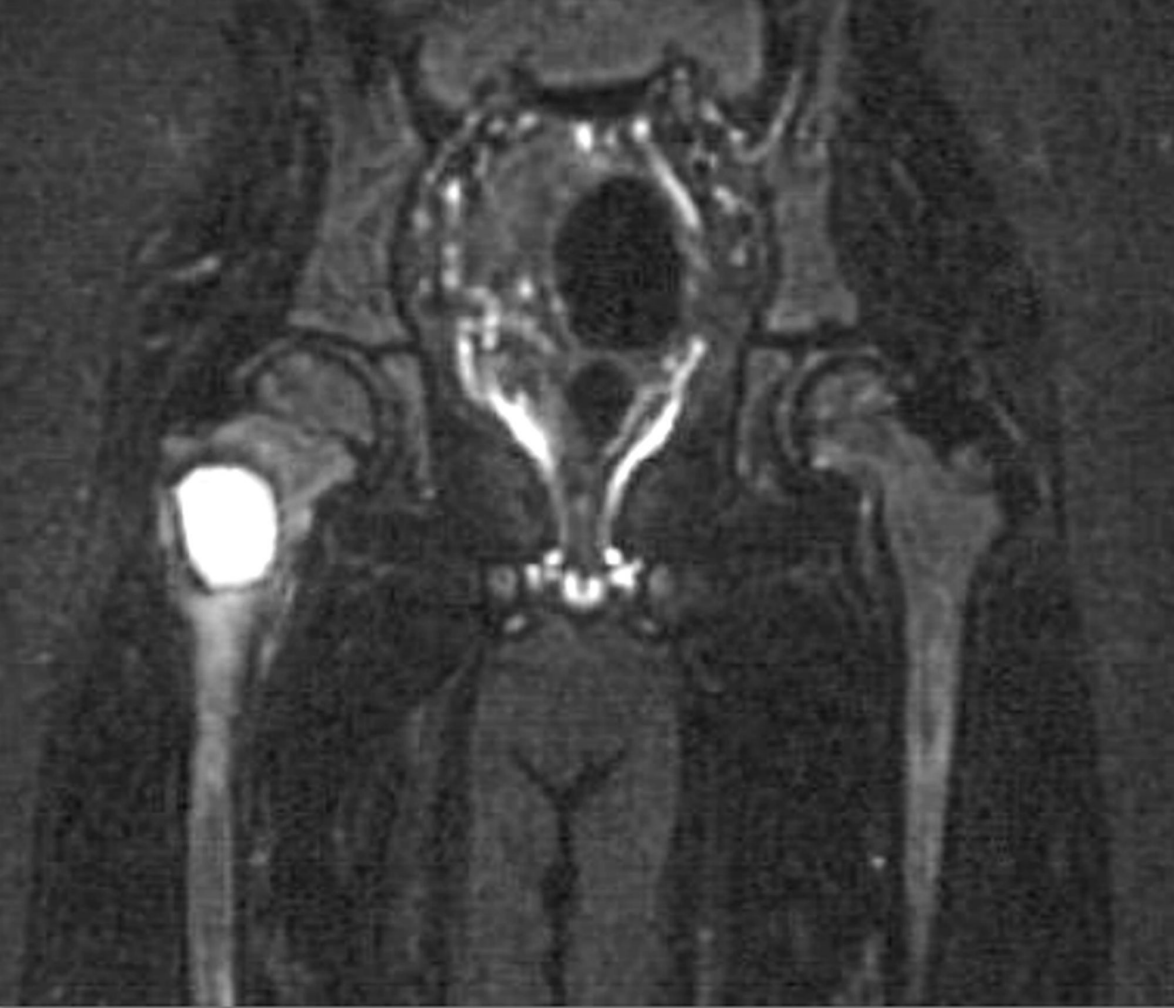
MRI T2-weighted section Shows a lytic lesion in the proximal portion of the right femur.
